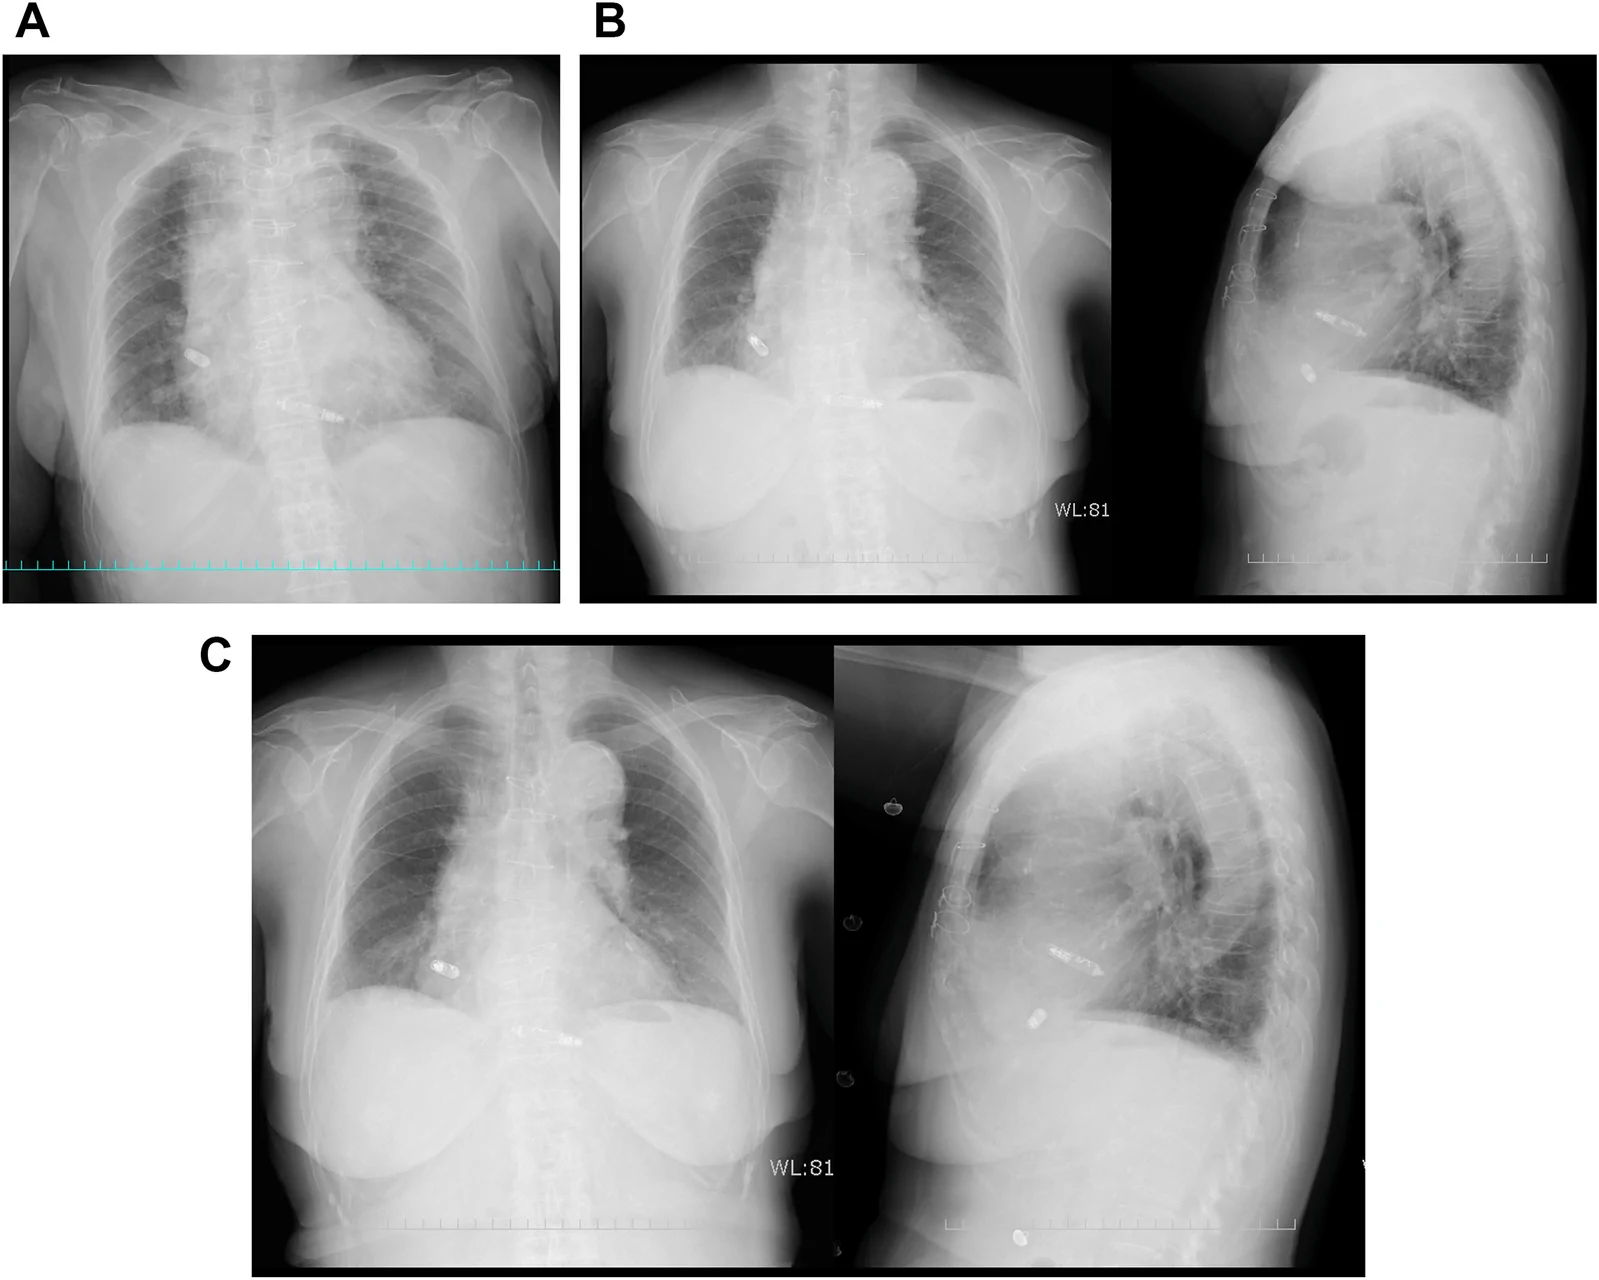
Chest radiographs in case 1. A: Implantation day. B: Postoperative day 1. Left panels show anteroposterior views, and right panels show lateral views. C: Postoperative day 13. Device position remained unchanged. Left panels show anteroposterior views, and right panels show lateral views.
